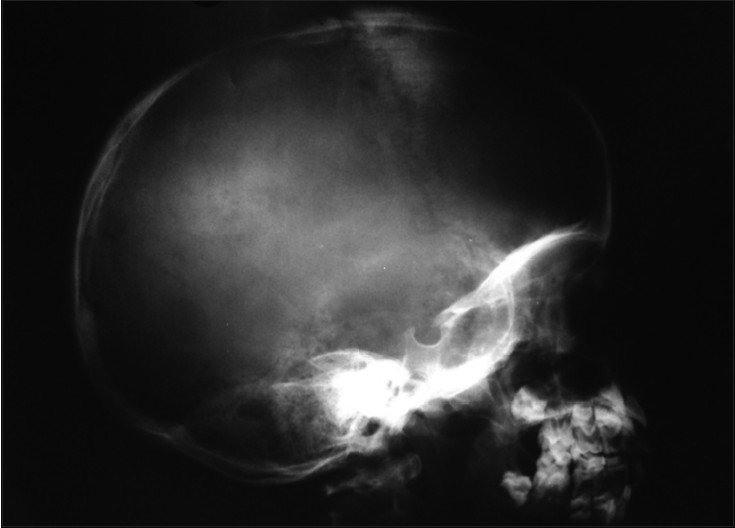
Post-treatment lateral radiograph of skull vault showing sclerosis in and around the lesion. The sclerosis seems to be bridging the diastasis
